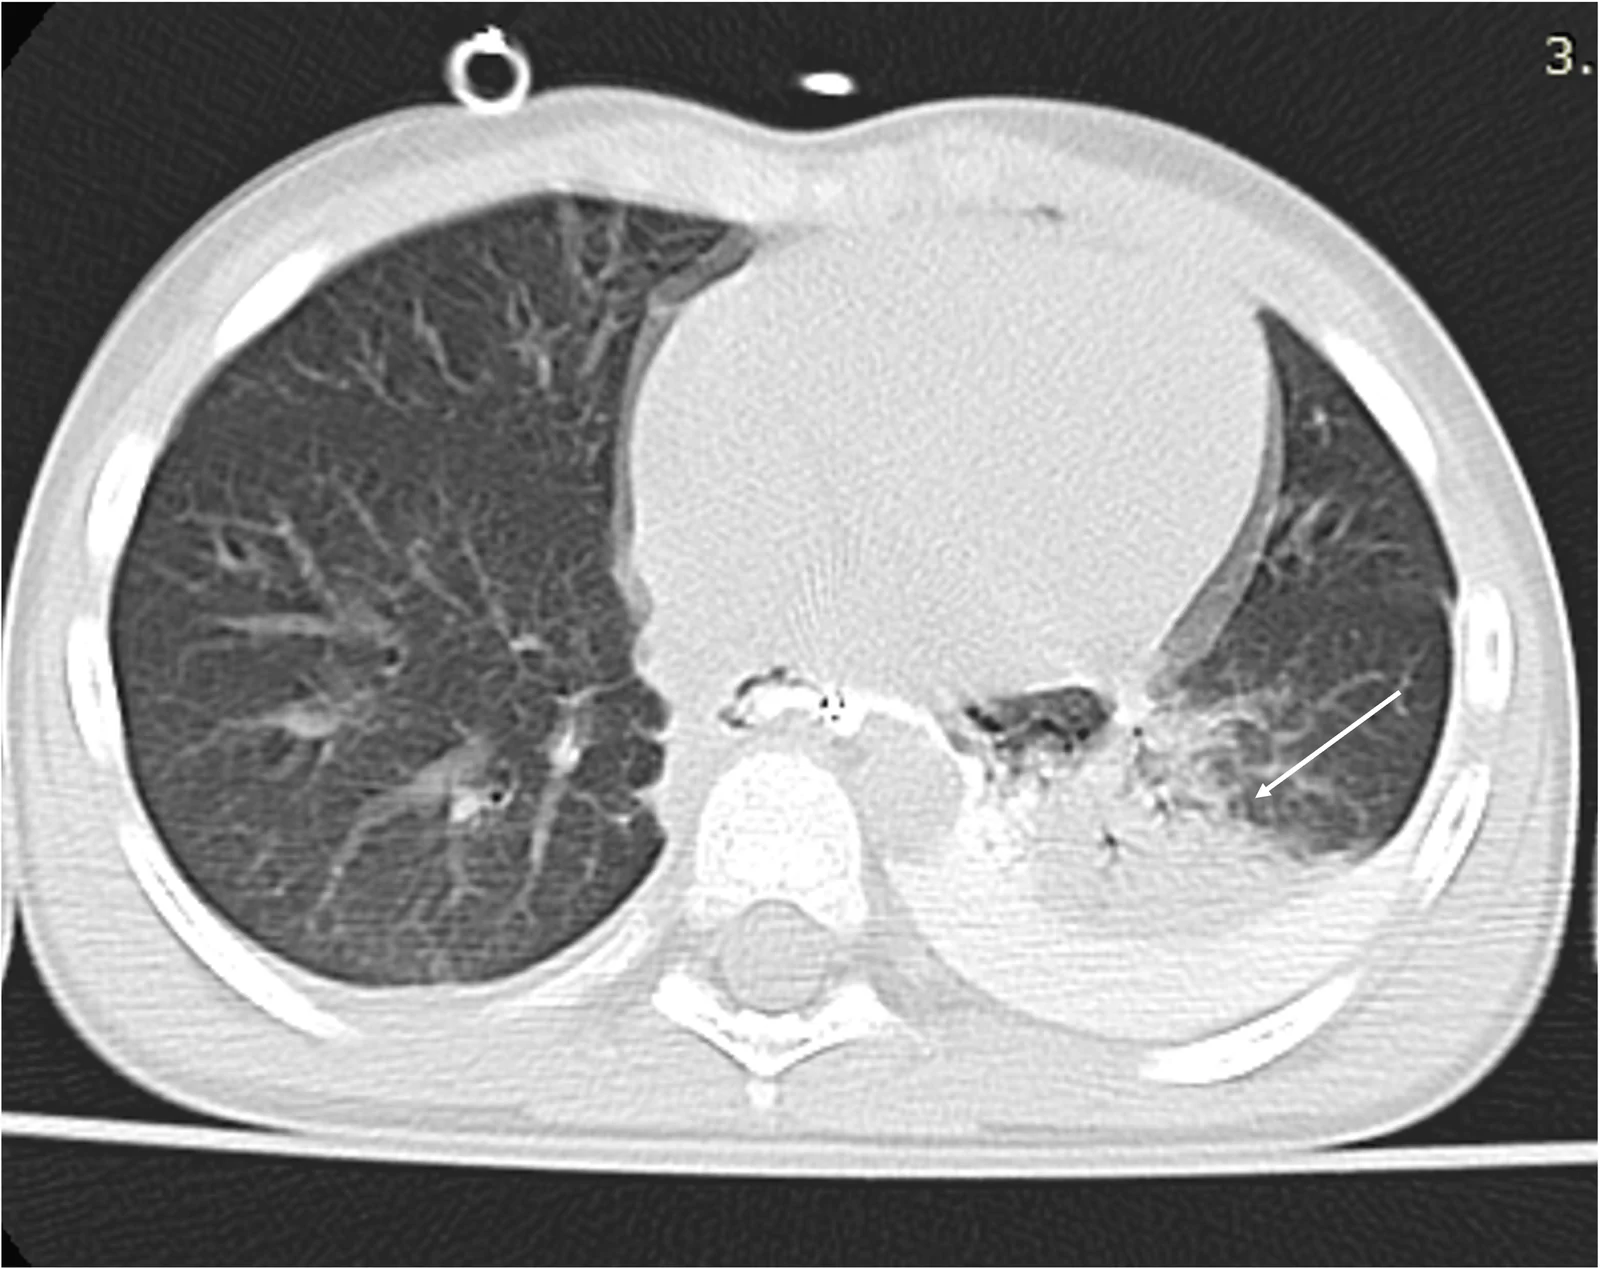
Axial thoracic CT scan showing a left pleural effusion and mediastinal inflammatory changes. The white arrow indicates the area of mediastinal inflammation adjacent to the distal esophageal rupture. CT: computed tomography
